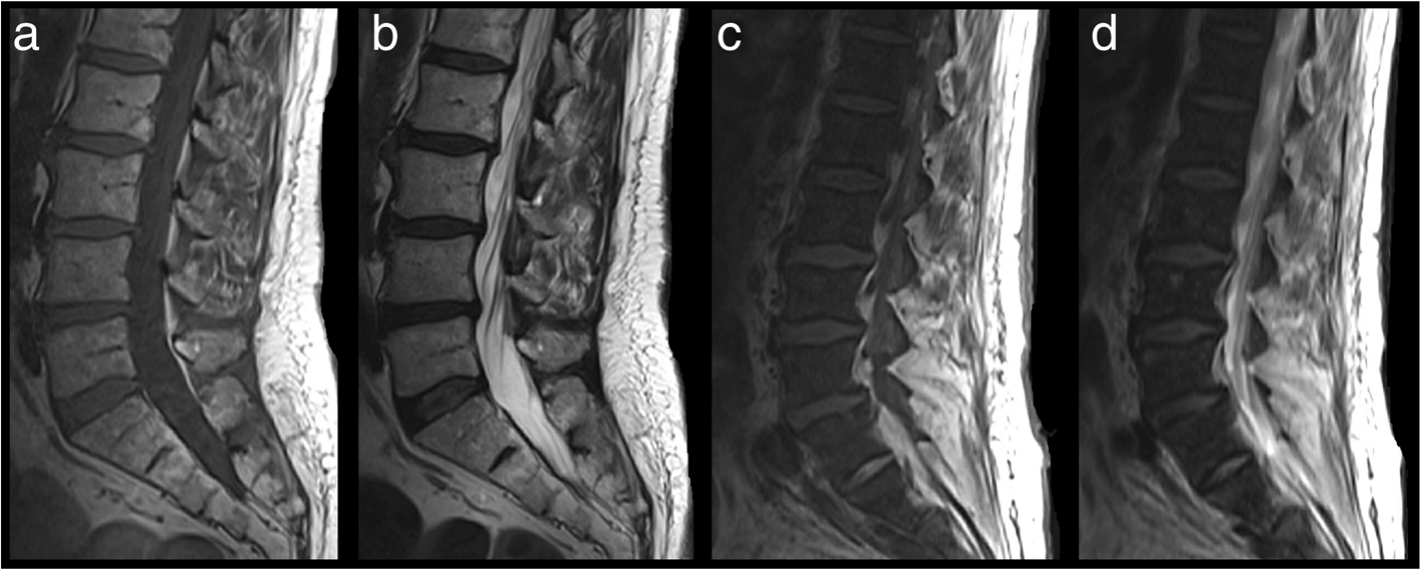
Sagittal T1 (a) and T2 (b) MRI of the lumbar spine in a normal patient demonstrating normal marrow appearances. The vertebrae are homogenous, and the intervertebral disks are hypointense relative to the marrow. In contrast, the marrow signal in myelofibrosis appears markedly hypointense on both T1 (c) and T2 (d). The intervertebral disks also appear hyperintense relative to the marrow signal
